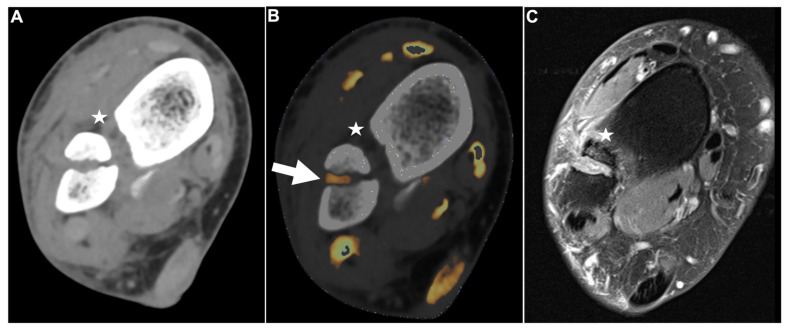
Fractured fibula with DTFS tear. Standard axial unenhanced grayscale CT (A), color-coded collagen reconstructions (B), and proton density-weighted unenhanced MRI series with fat saturation (C) of a fractured fibula with rupture of the ATIFL (stars). Notably, in the grayscale images, the ATIFL is visible (star); however, collagen mapping reveals no collagen signal, indicating a rupture. Note the distortion of the collagen signal by bleeding in the fractured region (B, arrow). MRI confirmed rupture of the ATIFL (star) and PTIFL (not pictured due to angulation). Abbreviations: ATIFL, anterior tibiofibular ligament. PTIFL, posterior tibiofibular ligament.
